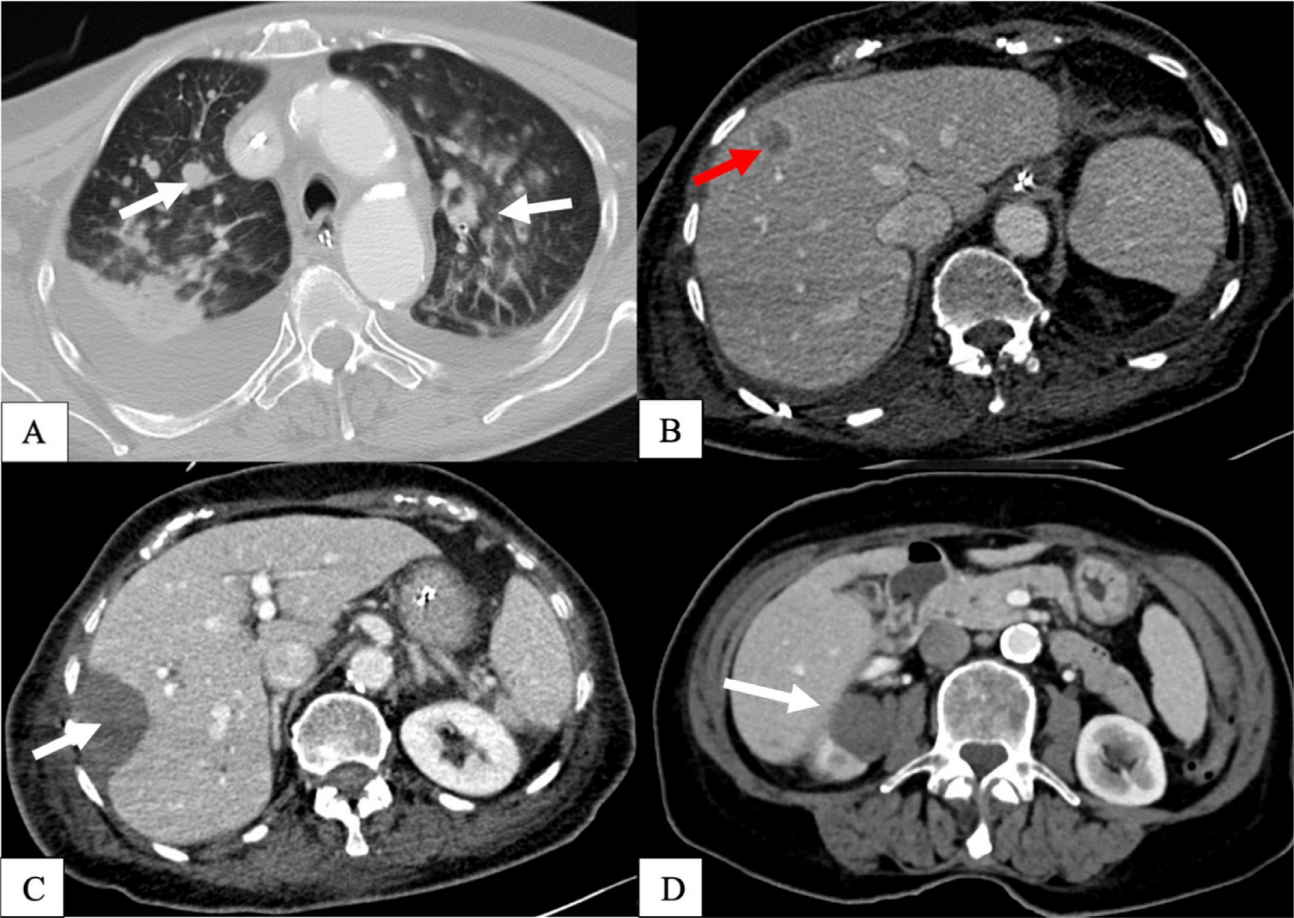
(A-D): Full-body CT scan 3 months after discharge from the hospital. 7A and 7B (axial view of Chest CT and Abdomen-CT) reveal hypodense lesions in the lungs and hypodense lesions with rim enhancement in liver segment VIII measuring 17 mm and in liver segment V measuring 14 mm, corresponding to new metastases. 7C and D (axial view of Abdomen and Kidney-CT) show no local recurrence of metastases adjacent to the ablation zone.
